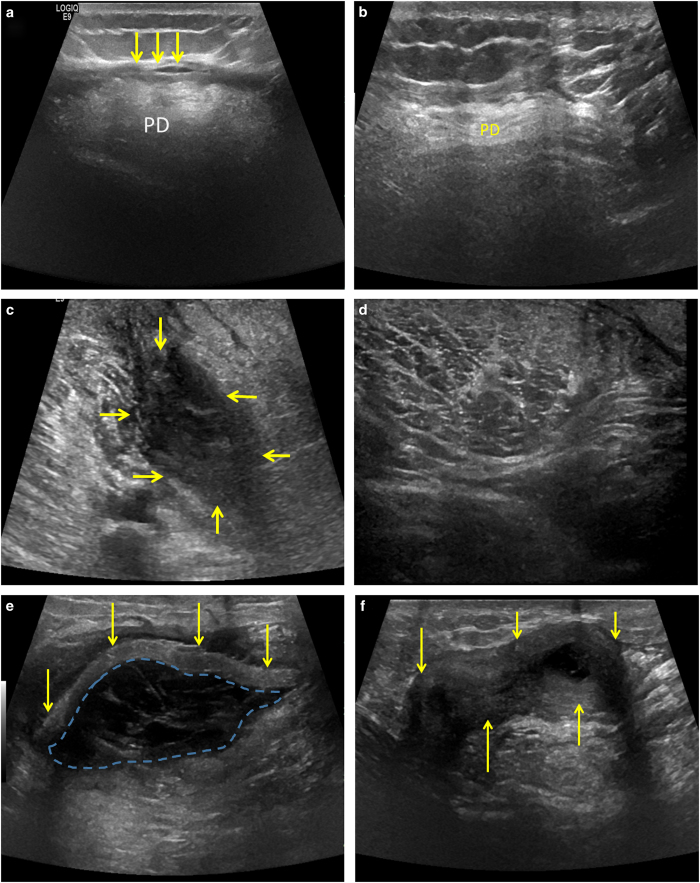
Ultrasound imaging shows that ECM bioscaffolds degrade on implantation. (a) Grayscale ultrasound image 1 month after surgery in the posterior shoulder demonstrates a thin, sheet-like hyperechoic structure representing SIS-ECM (yellow arrows) overlying the posterior deltoid muscle. The posterior deltoid muscle is increased in echogenicity due to underlying fatty infiltration. (b) Ultrasound imaging 7 months after surgery shows that surgically-placed SIS-ECM is not longer identifiable superficial to the posterior deltoid. (c). Ultrasound image 1 month after surgery in the medial mid thigh demonstrates an ill-defined hypoechoic structure representing SIS-ECM (yellow arrows) adjacent to the sartorius muscle. (d) Ultrasound image 7 months after surgery shows that surgically-placed SIS-ECM is no longer identifiable and the sartorius muscle appears to have enlarged. (e) Ultrasound imaging 1 month after surgery in the posterior mid thigh demonstrates a sheet-like echogenic structure representing dermal ECM (yellow arrows) with surrounding complex anechoic material (dashed-blue line) likely representing post-operative fluid collection. (f) Ultrasound imaging 7 months after surgery shows dermal ECM (yellow arrows) has decreased in echogenicity and now has a tubular or ‘rolled-up’ appearance as opposed to a sheet-like appearance. The previously identified post-operative fluid collection has essentially resolved.
